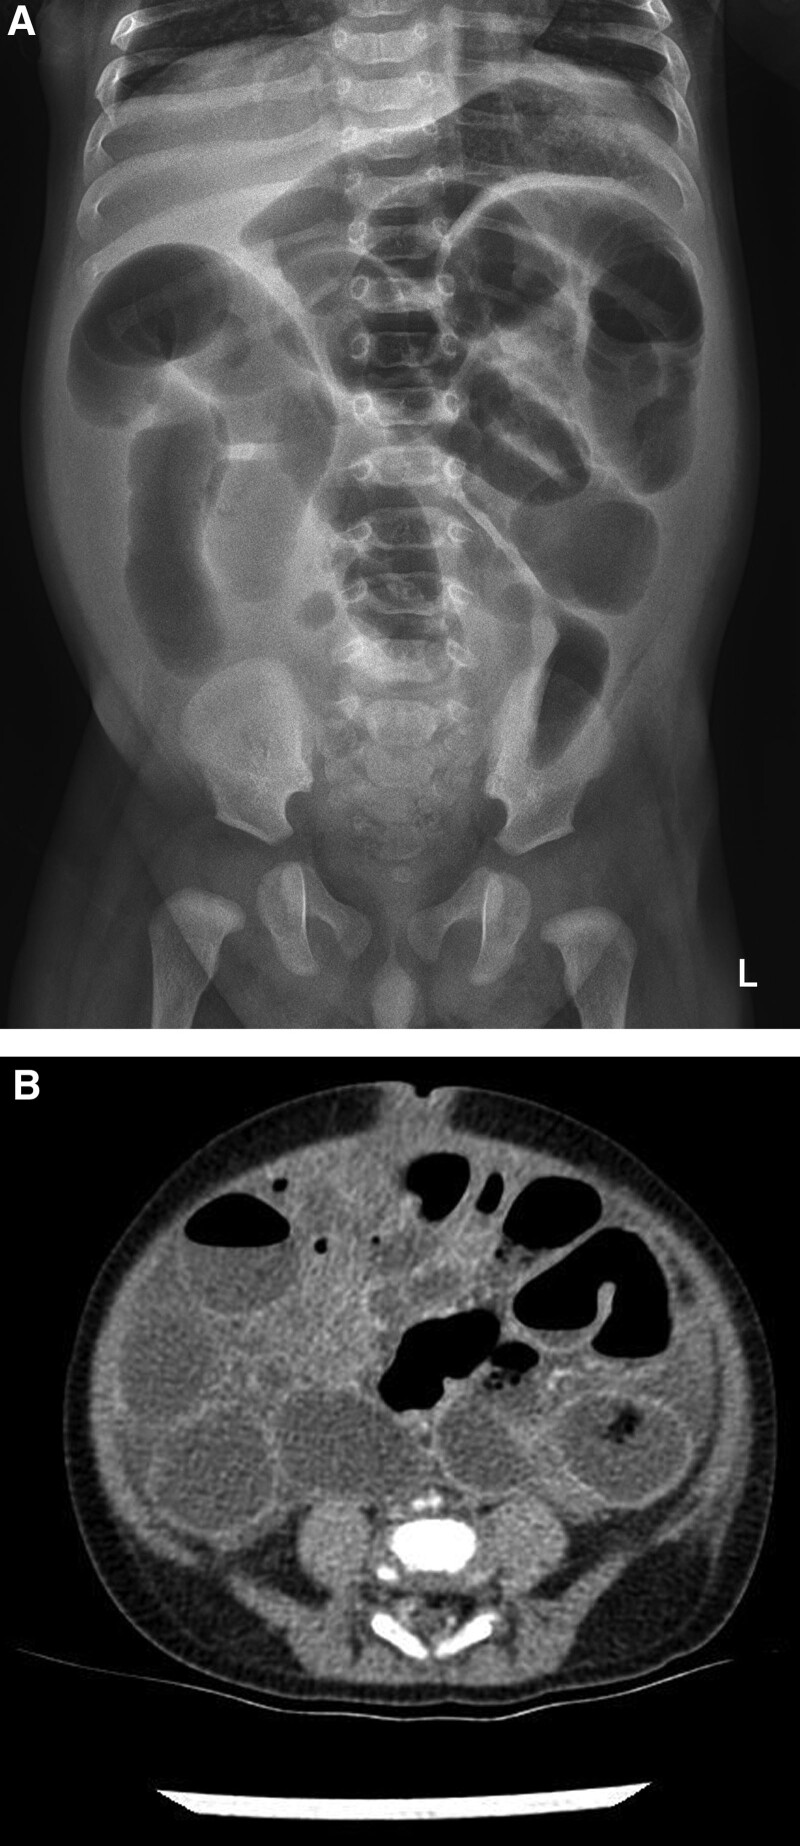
(A) Follow-up X-ray of the abdomen demonstrating small bowel ileus, and (B) computed tomography showing an ill-defined soft tissue density measuring approximately 4.5 × 4.3 cm in the mid-abdomen with associated intestinal obstruction (Day 2).
